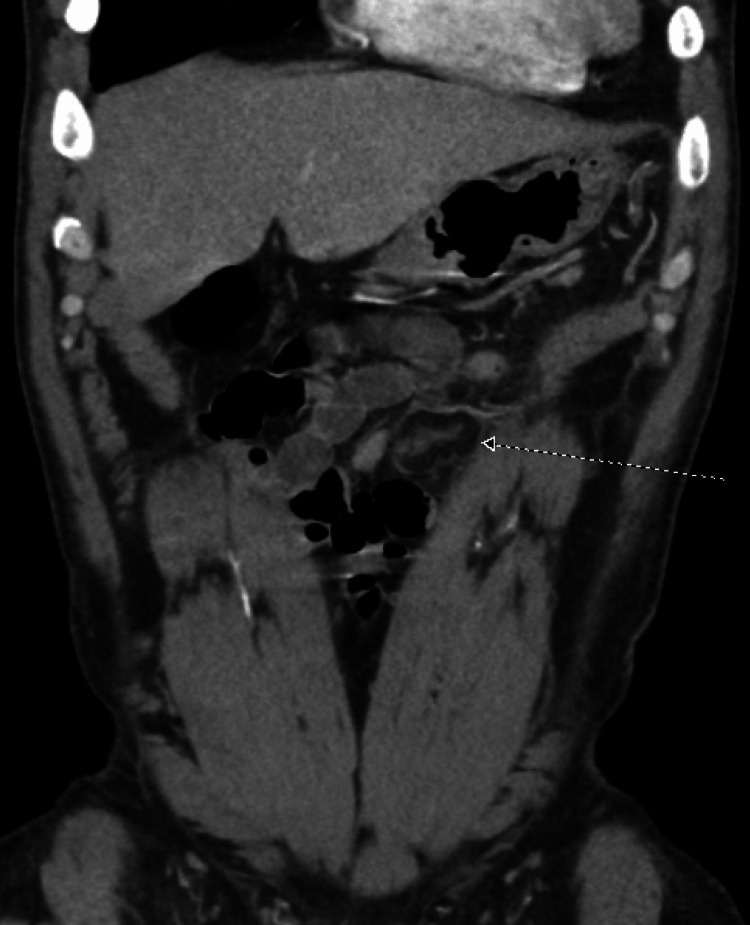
Computed tomography (CT) of the pelvis The arrow points to the 3.8 cm sharply circumscribed fat attenuation structure in coronal view. This is most consistent with epiploic appendagitis.
